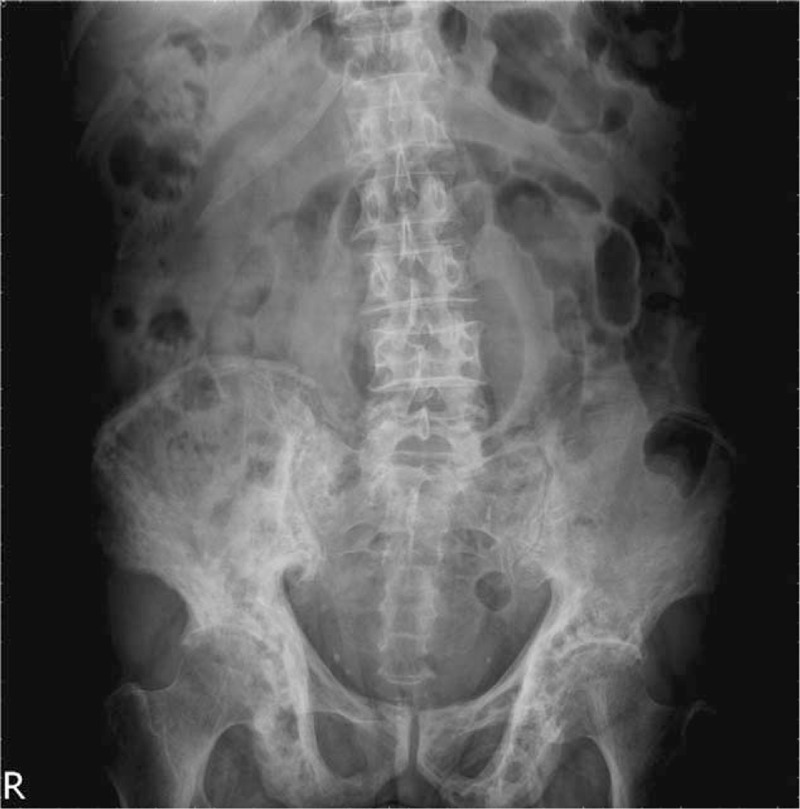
The abdomen plain film with mixed osteoblastic and osteolytic changes in the pelvic bone.
